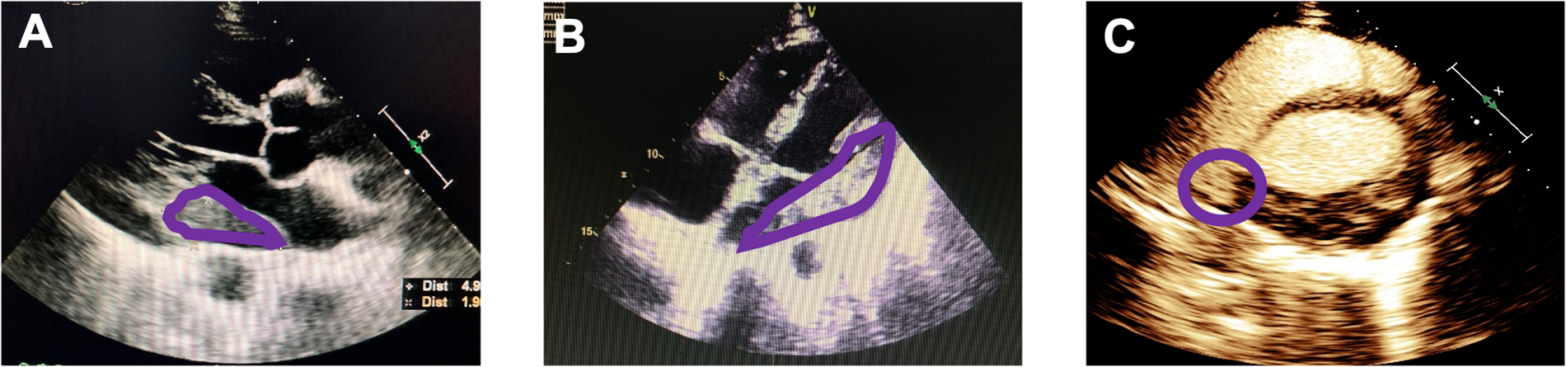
Echocardiography imaging: (A) parasternal long axis section; (B) four-chamber section. An intracardiac echogenic mass (in the purple circles) 50 mm × 67 mm × 19 mm was visualized at the left atrioventricular junction outside the cardiac cavity. (C) Left ventriculography and myocardial contrast echocardiography showed an abundance of contrast agent filling into left ventricular and the mass.
